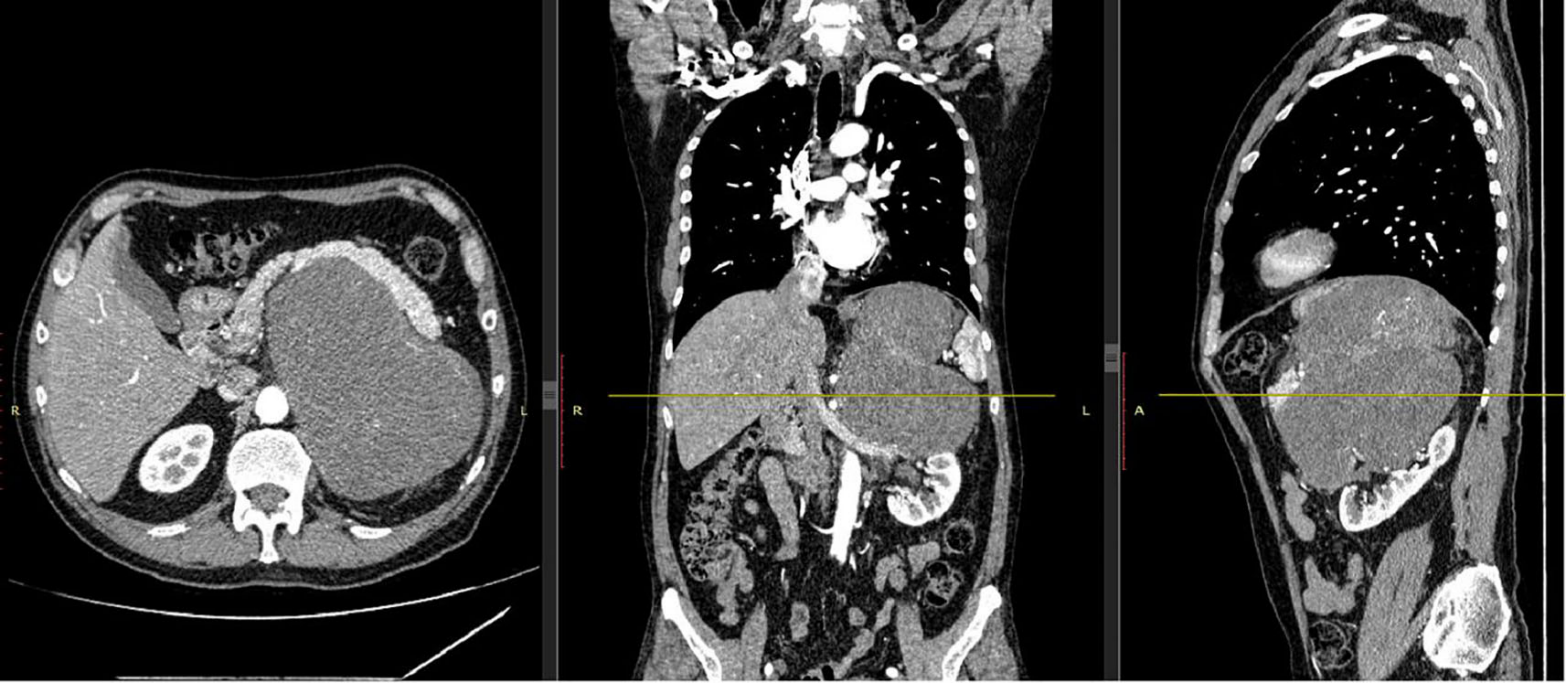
CT imaging of a retroperitoneal mass with mixed density.
